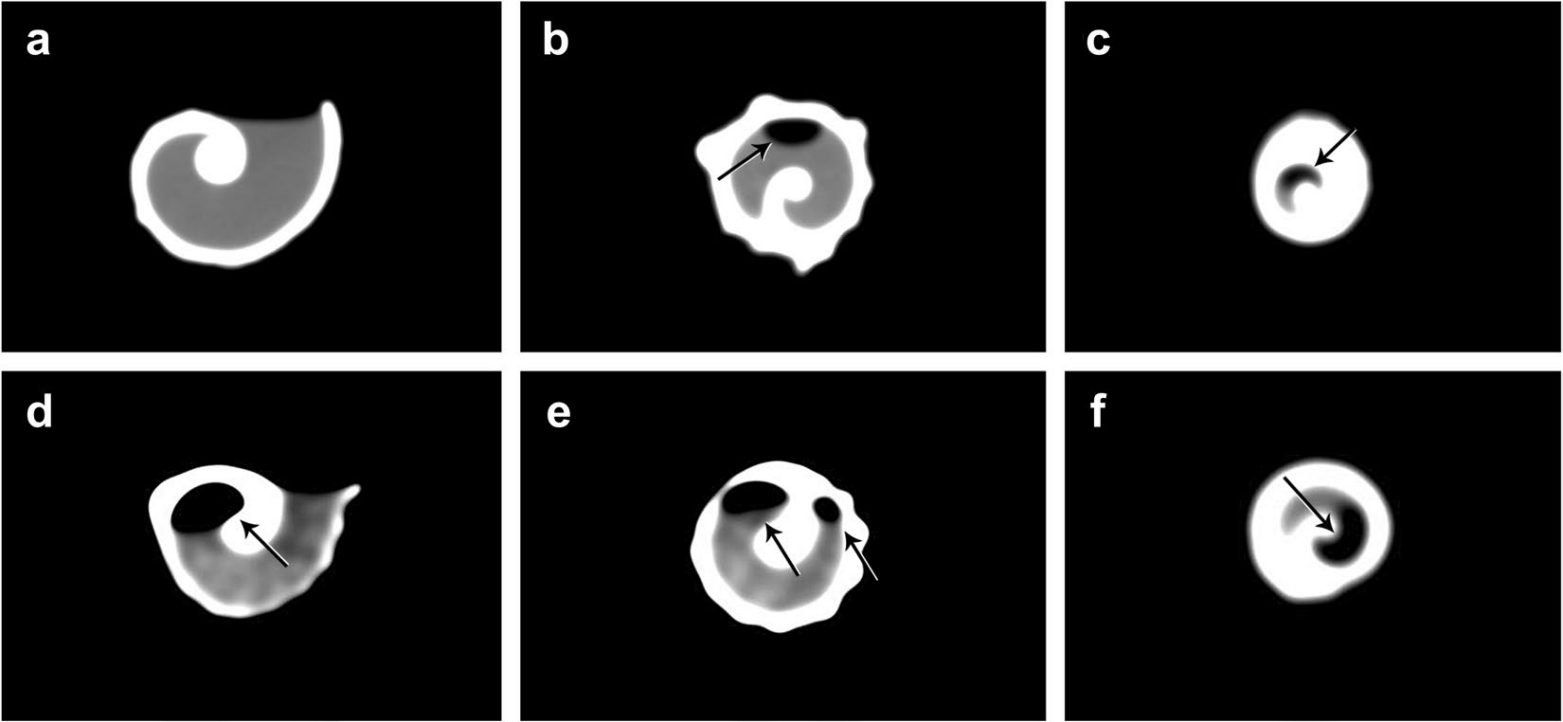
Computed Tomography slices of single gastropod shells filled with water (*Stramonita haemastoma*; (a) body whorl, (b) mid shell and (c) shell apex) and sand (*Cymatium parthenopeum*; (d) body whorl, (e) mid shell and (f) shell apex). The filled portion of the shell internal space is represented in gray, while the air spaces are represented in black (indicated by arrow). Note that the shell apex is not totally filled using either methods (c,f).
